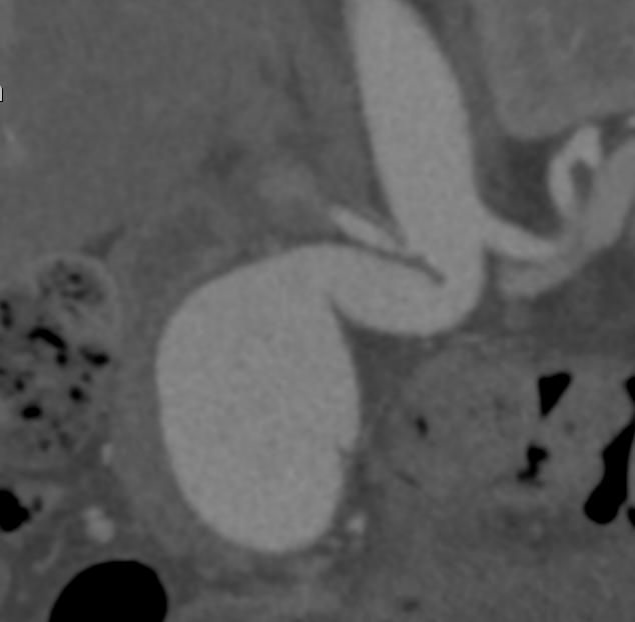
Preoperative CT angiography image showing tortuous aortic neck.
